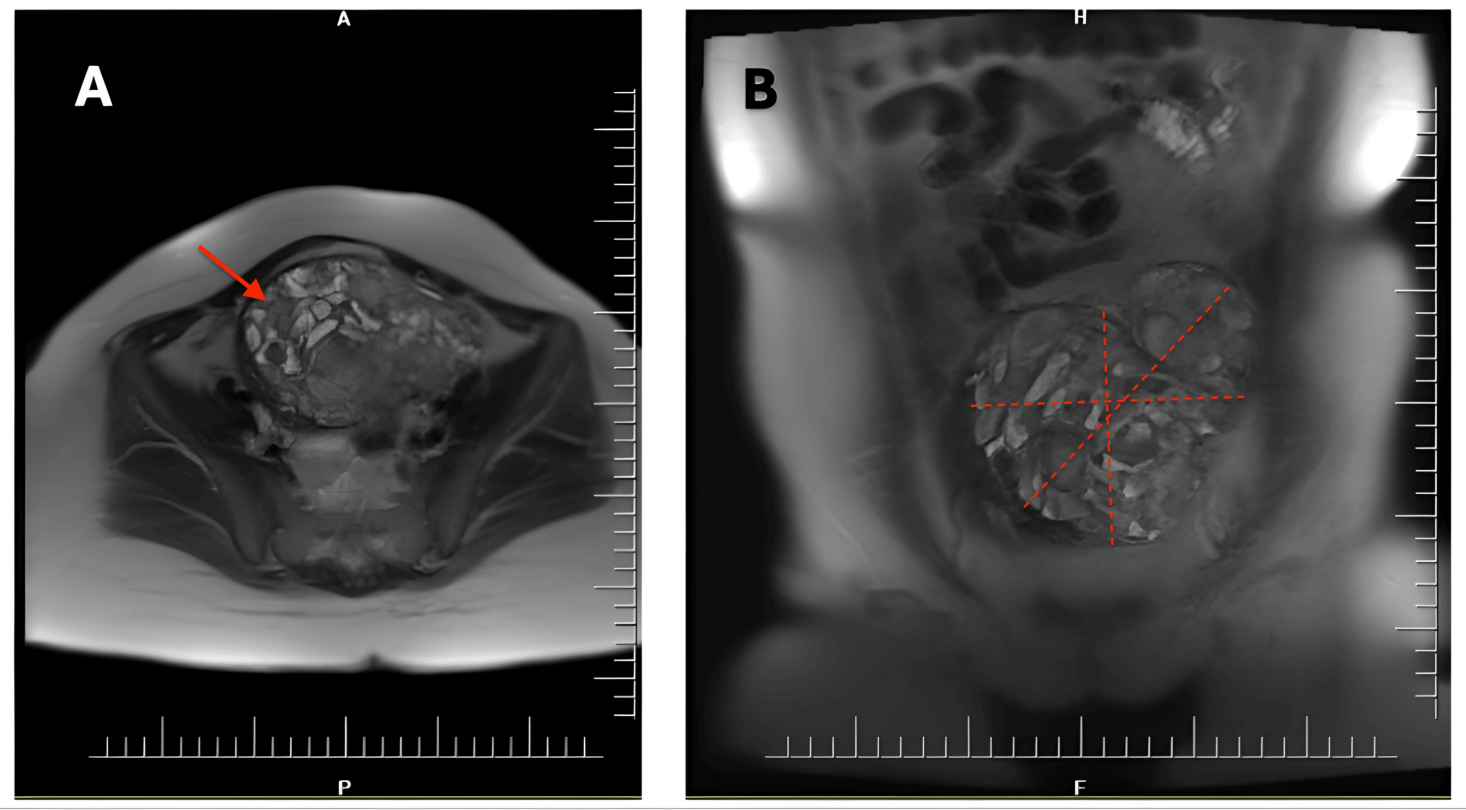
Contrast-enhanced magnetic resonance imaging of the abdomen and pelvis. A. Axial section showing a lesion with diffuse infiltration of the myometrium and endometrium, with multiple cystic-appearing images and vascular malformations. B. Coronal section showing marked restriction on diffusion-weighted sequences, measuring 14 cm in longitudinal diameter, 10 cm in anteroposterior diameter, and 12 cm in transverse diameter. Image provided from the patient’s medical records with prior authorization.
